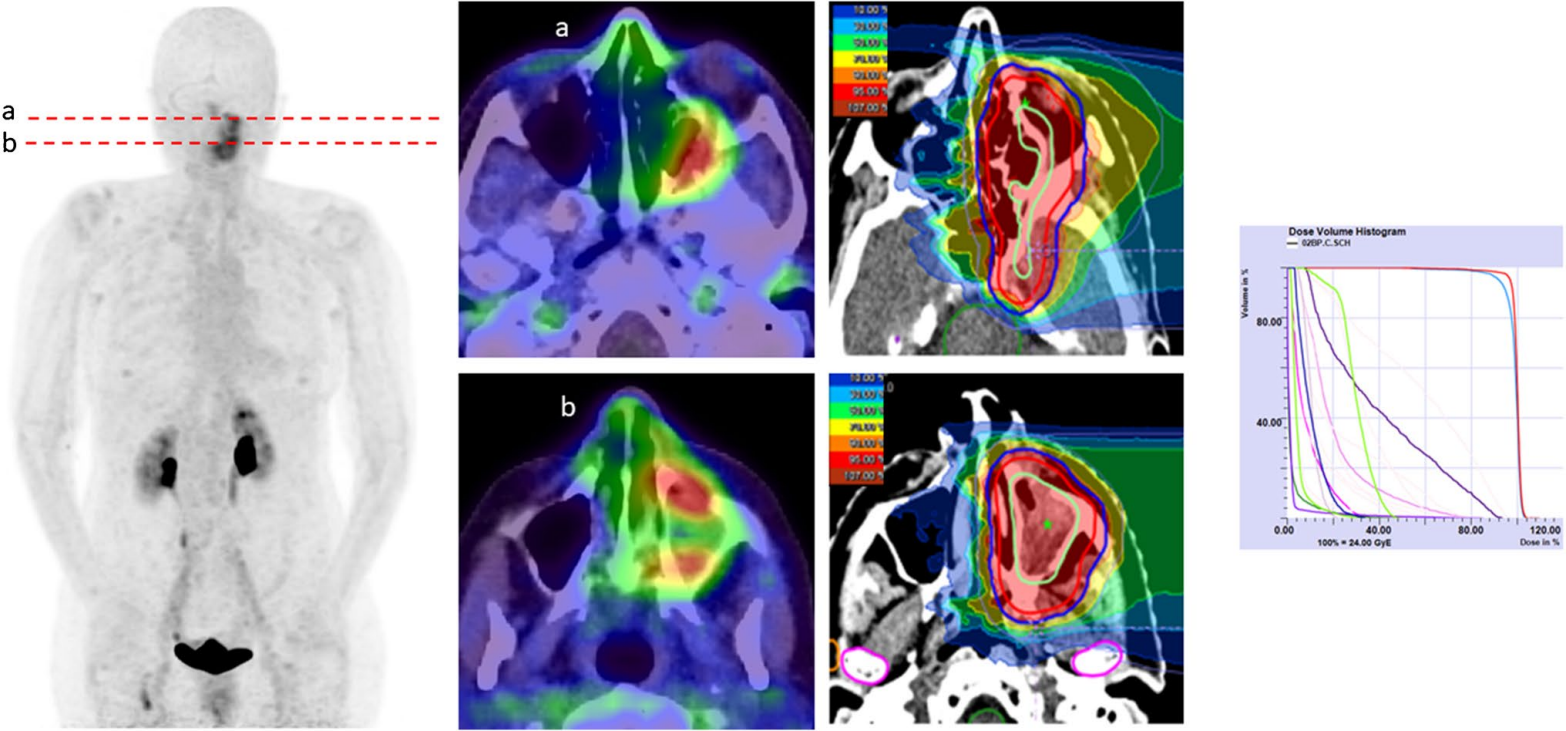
A 71-year-old female patient with HPV-associated adenoid cystic carcinoma (SUVmax 6.7) located in the left fossa pterygopalatine underwent ^68^ Ga-FAPI-PET/CT for radiotherapy planning
